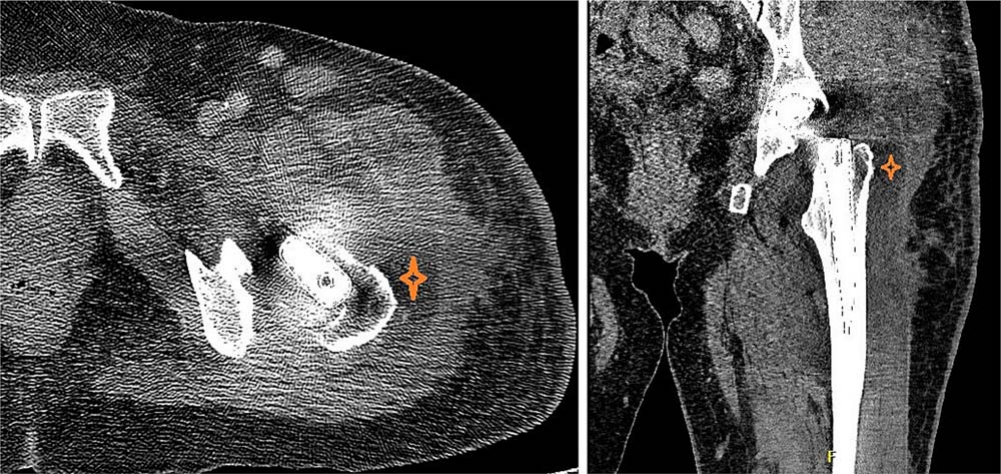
CT of the hip with contrast showed peripherally enhancing deep fluid collection (marked with star) containing gas pockets abutting the left proximal femur extending to the subcutaneous tissue and the gluteus medius muscles and measuring 7.2 × 6.6 × 14 cm with overlying skin thickening and fat stranding.
